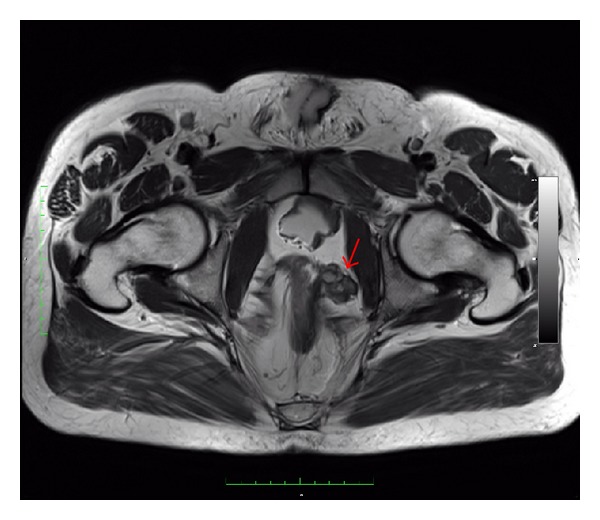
Pelvic MRI of the patient. The arrow demonstrates the pararectal GIST.
